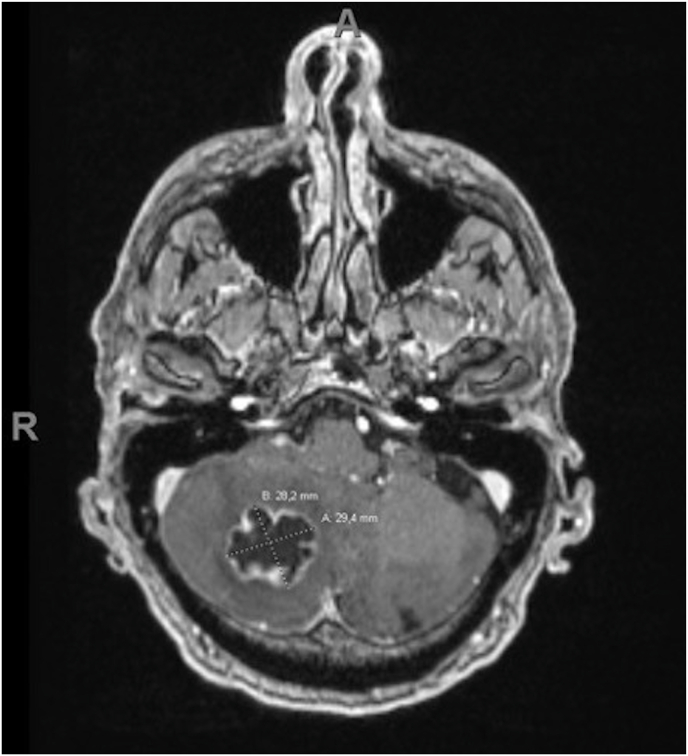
T1-weighted gadolinium-enhanced transverse image of MRI brain examination. The metastatic lesion is located in the right cerebellar hemisphere obstructing the fourth ventricle without supratentorial hydrocephalus.
